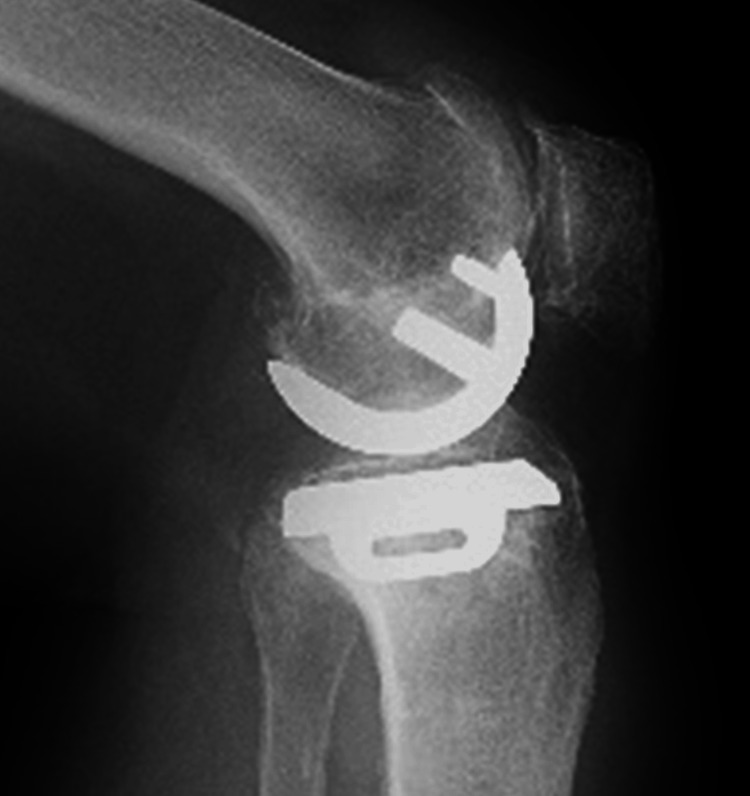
Radiography of refracture after plate removal (lateral view)
